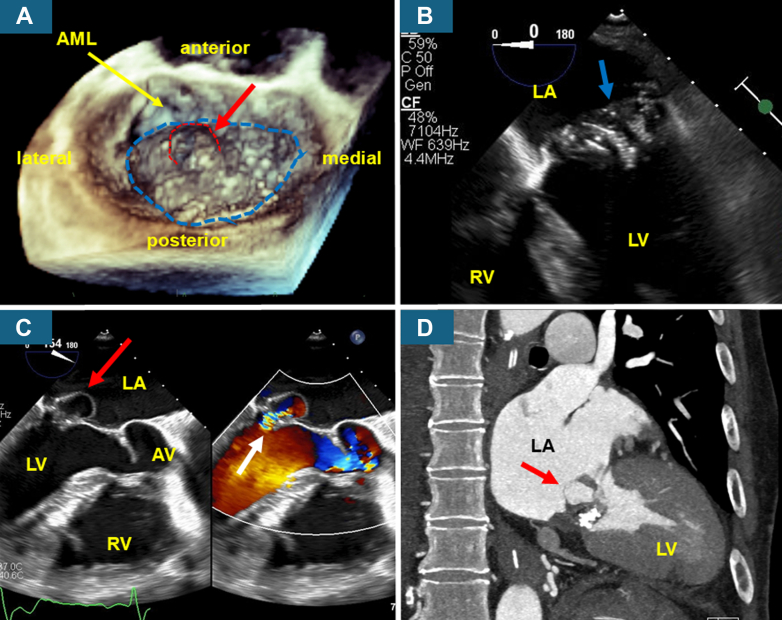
Multimodal Imaging Demonstrating Mitral Valve Vegetation With Aneurysm (A) Three-dimensional transesophageal echocardiography showing a broad, flat, disc-shaped vegetation (blue dashed line) with an overlying cystic structure (red arrow and red dashed line) covering the atrial surface of both the anterior and posterior mitral leaflet. (B) Two-dimensional transesophageal echocardiography midesophageal 4-chamber view revealed vegetation with substantial thickness deposited on and adherent to the mitral valve (blue arrow). (C) Color Doppler imaging long-axis view demonstrated systolic inflow (white arrow) from the left ventricular side into an associated cystic structure (red arrow), consistent with a mitral valve aneurysm. (D) Computed tomography revealed a cystic structure (red arrow) associated with the calcified mitral valve, showing contrast enhancement within the cavity. AML = anterior mitral leaflet; AV = aortic valve; LA = left atrium; LV = left ventricle; RV = right ventricle.
